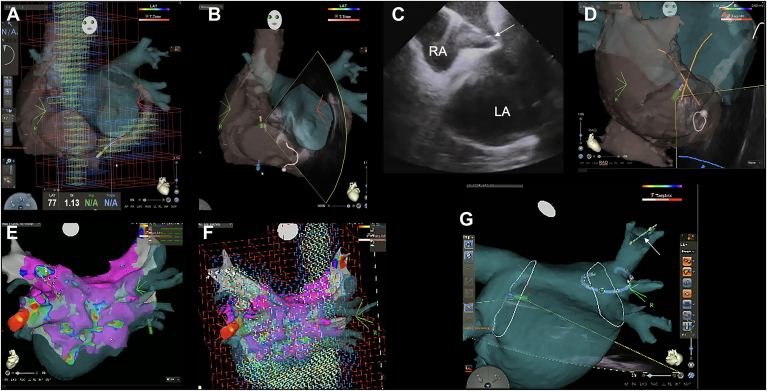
CARTO-guided workflow for near-zero fluoroscopy PFA using the PulseSelect catheter. A: Matrix of the RA, SVC, IVC, and CS, constructed using an OCTARAY catheter without fluoroscopy. B: CARTO 3D image showing the SOUNDSTAR catheter during transseptal puncture. The ultrasound fan is displayed to assist in confirming catheter orientation and safe passage through the septum. C: ICE image during transseptal puncture using an ACCUSAFE needle (*white arrow*). D: CARTO 3D visualization of the interatrial septum. A cross (“×”) mark indicates the previously confirmed puncture site, and the SOUNDSTAR catheter tip is directed toward it and advanced into the LA. E: LA anatomic map acquired using an OCTARAY catheter. F: Same LA anatomy as in panel E, shown with matrix display enabled to confirm adequate mapping coverage prior to ablation. G: Visualization of the wire tip during PFA. The wire is connected with an alligator clip to render its position visible on CARTO; the tip (*white arrow*) is advanced into the RSPV under 3D guidance. CS = coronary sinus; ICE = intracardiac echocardiography; IVC = inferior vena cava; LA = left atrium; PFA = pulsed field ablation; RA = right atrium; RSPV = right superior pulmonary vein; SVC = superior vena cava; 3D = 3-dimensional.
